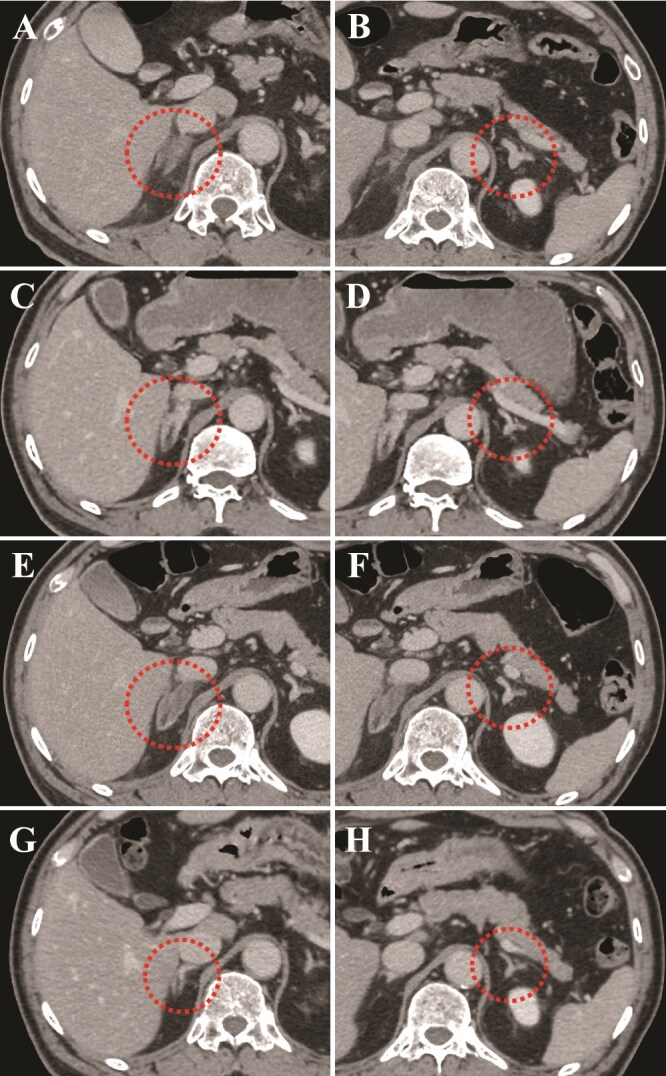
Axial section images of contrast-enhanced computed tomography scan. The left images (A, C, E, and G) show the right adrenal gland, and the right images (B, D, F, and H) show the left adrenal gland. (A and B) day 0: Bilateral enlarged adrenal glands with no enhancement after contrast injection. (C and D) day 8: Improvement in size and contrast-enhanced effect after sufficient pain control. (E and F) day 9: Bilateral enlarged adrenal glands and poor enhancement at the time of new left abdominal pain. (G and H) three months later: Bilateral adrenal glands normalized in both size and contrast-enhanced effect.
